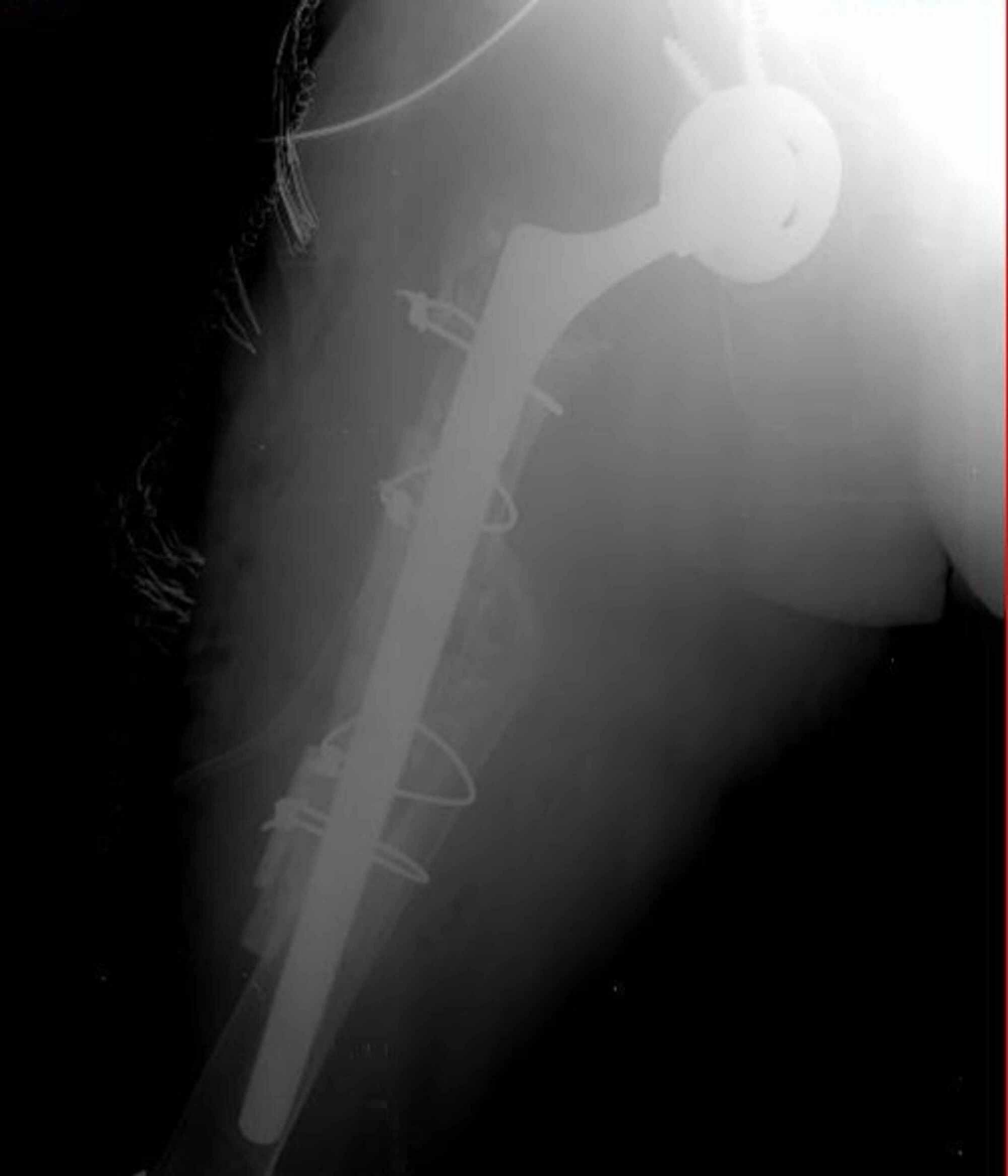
Early postoperative radiography of the patient who underwent revision total hip replacement.
